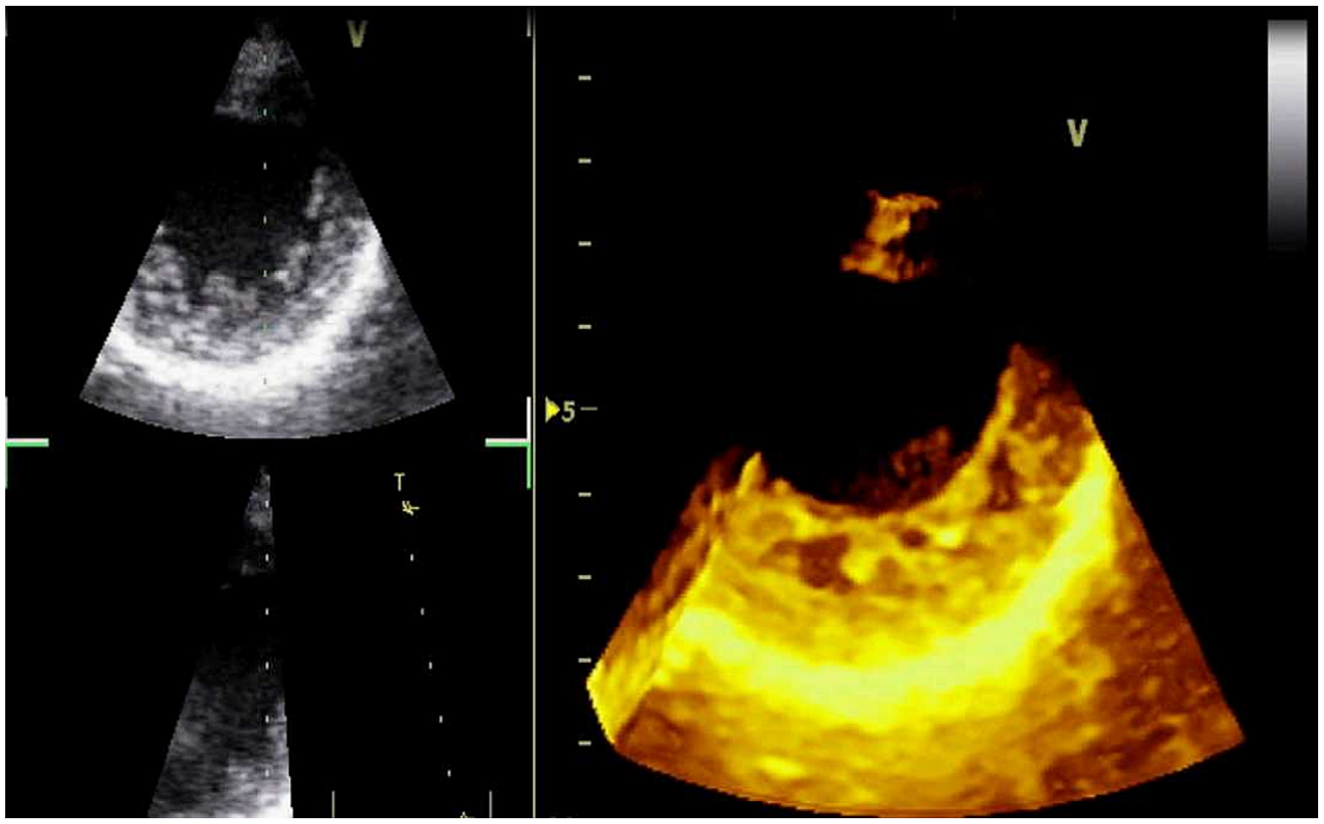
Real-time three-dimensional echocardiographic appearance from parasternal short-axis view of a patient with isolated left ventricular noncompaction.
